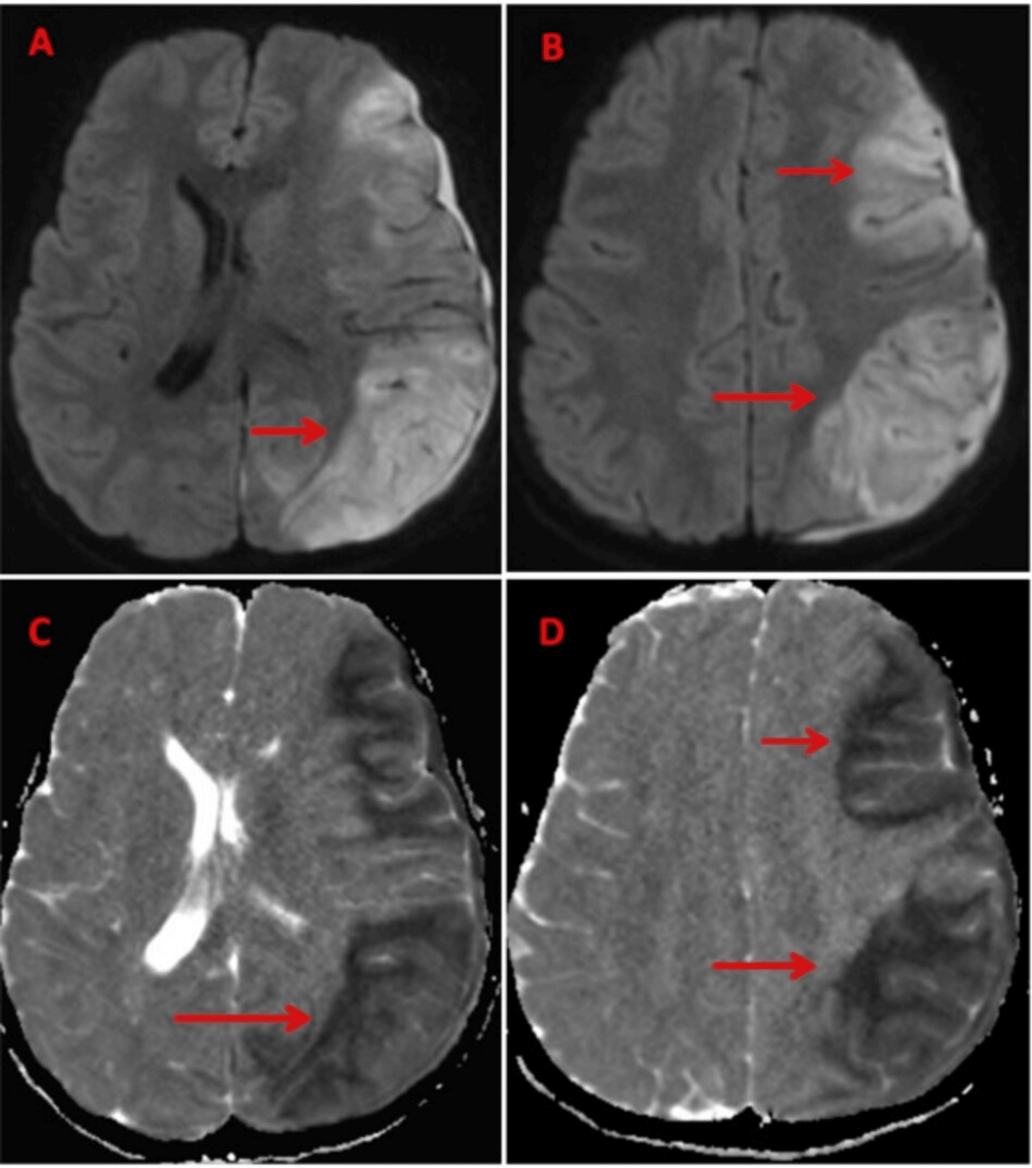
DWI (A-B) and ADC (C-D) showing a non-hemorrhagic infarct in the left fronto-parietal region, appearing bright on DWI with low ADC values (marked by red arrow). DWI: diffusion-weighted imaging, ADC: apparent diffusion coefficient.
